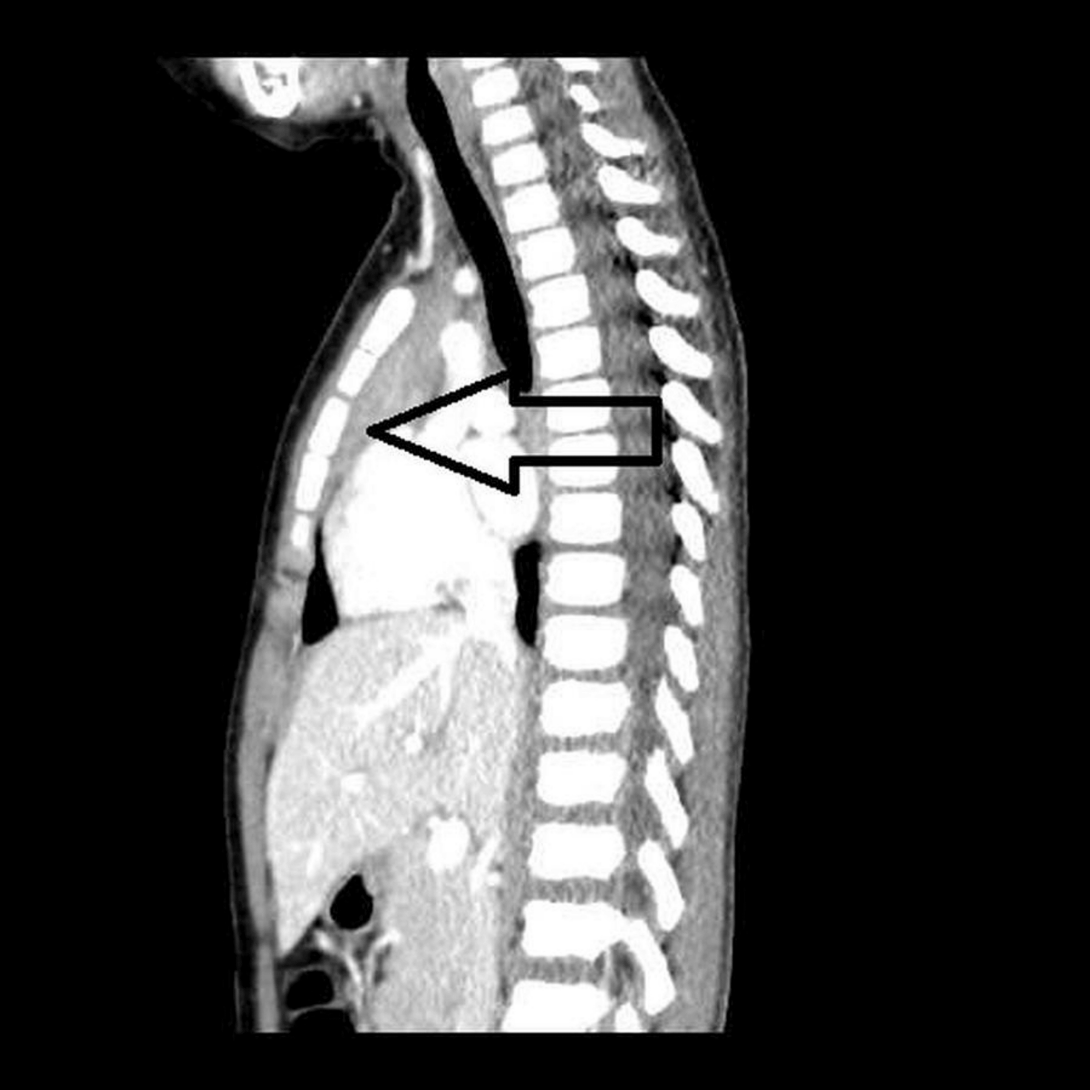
Sagittal chest CT showing absence of dislocation and normal mediastinal anatomy CT: computed tomography
